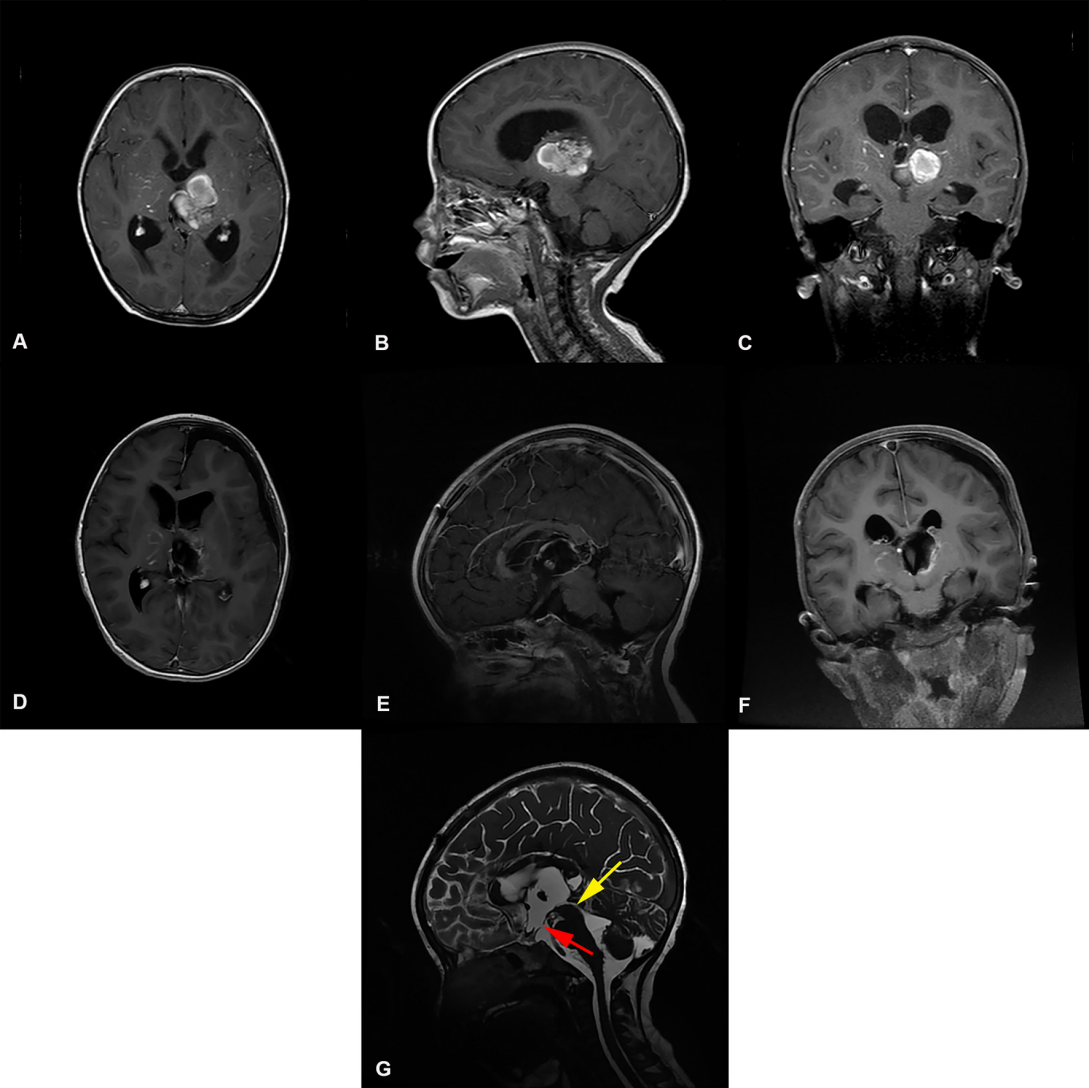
Preoperative and postoperative imaging of thalamic cavernous hemangioma resection with left trans-frontal lateral ventricle approach + third ventriculostomy. (A-C) display: Axial, sagittal and coronal enhanced MRI of benign tumors of the left thalamus. The MRI shows that the main body of the tumor was located in the left thalamus, invading the third ventricle, and the supratentorial lateral ventricle and third ventricle were dilated. (D-F) display: MRI images of thalamic tumor resection with left trans-frontal lateral ventricle approach + third ventriculostomy. The images show total resection of the tumor; the volume of the lateral ventricle after surgical resection of the thalamic lesion did not expand. (G) display: Three ventriculostomy was performed after resection of thalamic tumor The MRI T2 shows that the upper mouth of the midbrain aqueduct was thin, as indicated by the yellow arrow(
